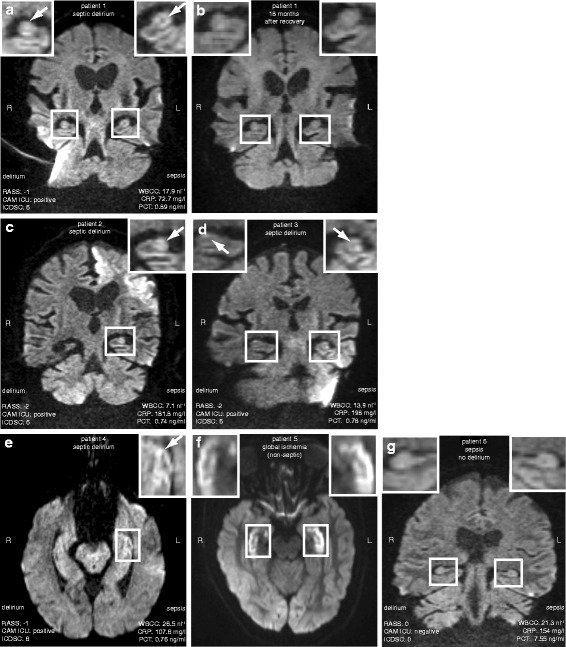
Pathologic signal changes in diffusion-weighted magnetic resonance imaging (DWI MRI) of the hippocampal formation in sepsis-induced delirium in human patients. a, b, c, d, e Images show coronal (a, b, patient 1 and c, patient 2, d, patient 3) and horizontal (e, patient 4) diffusion-weighted MRI scans of patients with sepsis and sepsis-induced delirium. Scores for delirium and sepsis shown in (panels a, c, d, e and g) were obtained within 24 h from the MRI scan. Boxed areas of the hippocampal formation are shown on an enlarged view in the top of each panel. White arrows indicate localized hyperintense signal elevations. b A scan taken from patient 1 eighteen months later shows no hyperintensities. The broad signal elevation in the left temporal lobe of patient 2 (c) was due to a previous occlusion of the left medial cerebral artery. f A scan from a non-septic patient with global cerebral hypoxia exhibits bilateral and broad signal elevation observed in the hippocampal formation. g Hippocampal hyperintensities were absent in septic patient without delirium. Hyperintense movement artifacts (right cerebellar area in a, left cerebellar area in d) or susceptibility artifacts (left temporal bone area in f) are occasionally observed in the DWI MRI scans. L: left; R: right; RASS: Richmond agitation sedation scale; CAM ICU: Confusion assessment method for intensive care unit; ICDSC: Intensive care delirium screening checklist; WBCC: white blood cell count; CRP: c-reactive protein; PCT: procalcitonin
